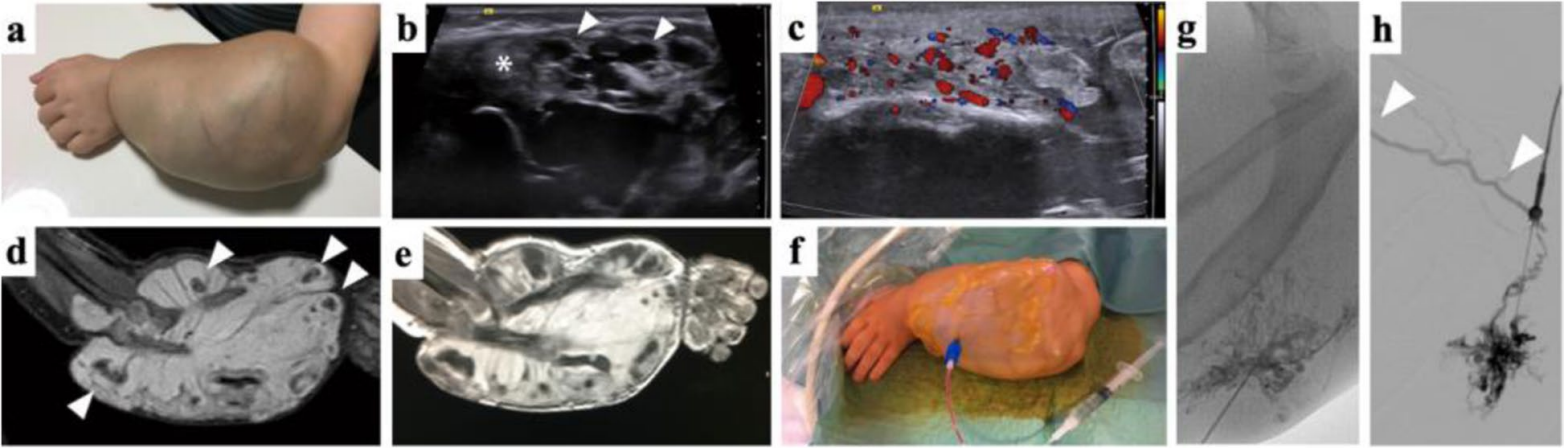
Venous Malformation a four-year-old child of the forearm with bone disfigurement. a Clinical presentation shows large, non-pulsatile painless soft tissue mass with bluish discoloration and visible dysplastic veins. Wrist function was restricted but finger motility was not significantly impaired. The heavy weight of the arm made the boy carry his left arm with the right hand. b B-Mode ultrasound imaging shows cavernous dysplastic veins (white arrowhead) and thrombosed parts (white asterisks) of the lesion. c Color-coded Duplex ultrasound reveals slow blood flow within the dysplastic vessels. d STIR MRI imaging shows typical hyperintense signal with multiple hypointense spots representing phleboliths (white arrowheads). T1-weighted MRI imaging after contrast administration shows enhancement of the large venous cavities of the VM (e). Venous malformations are treated by percutaneous sclerotherapy. The periprocedural image (f) shows confirmation of blood aspiration after percutaneous access to the dysplastic vessels, before using a contrast agent and a sclerosing agent subsequently. g Angiographic depiction of VM after contrast agent administration via percutaneous access displays a fine reticular network of dysplastic vessels and drainage to deep veins (h) not visible on MRI
